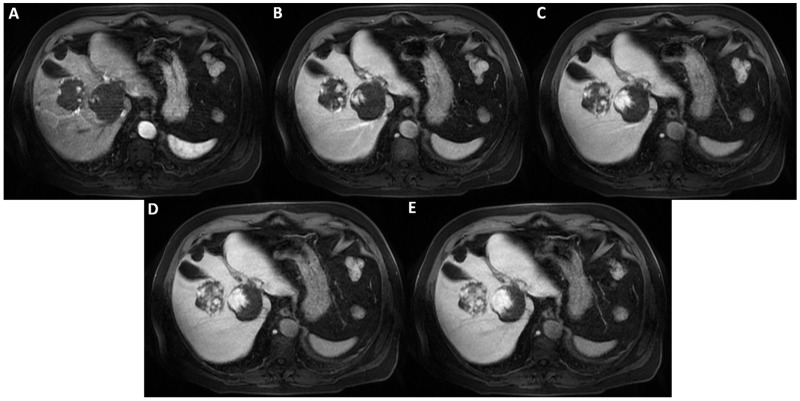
Axial T1-weighted fat saturated images after hepatospecific contrast agent (Gd-EOB-DTPA) administration in arterial, portal-venous, and delayed phases (A–E) show early peripheral discontinuous globular enhancement of the lesions with progressive centripetal filling-in on delayed scans. This angiodynamic behavior supports the diagnosis of cavernous hemangiomas.
